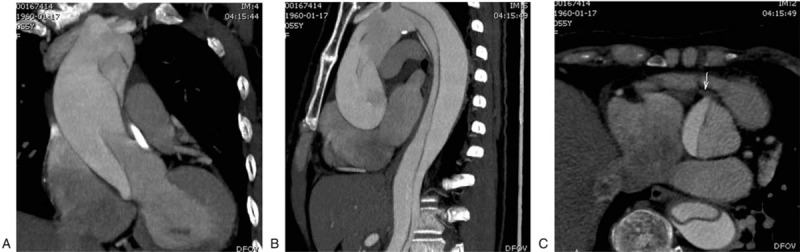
A and B: Multislice computed tomography reconstructions vividly showing aortic dissection from the aortic root to the abdominal aorta. C: Dissection violations of the right coronary artery ostium (arrows).
